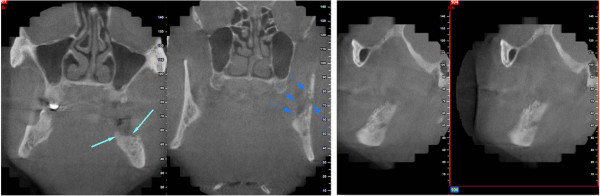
Coronal and sagittal cone beam computed tomography (CBCT) images showing erosion in the molar region through the temporomandibular joint (TMJ) area.
